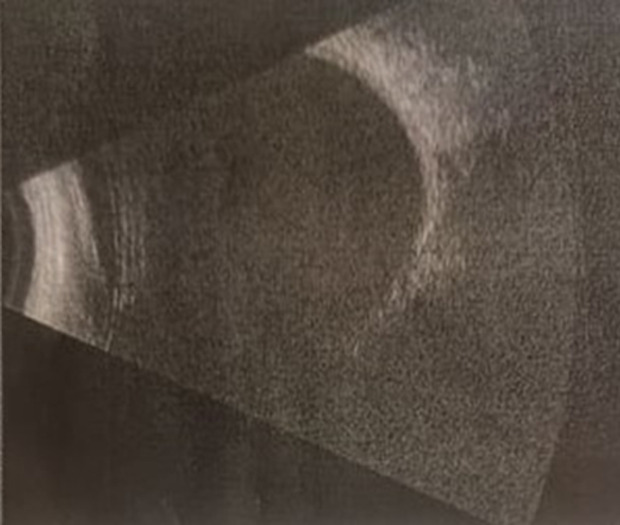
ultrasound B scan of the right eye showing a clear vitreous and no intraocular foreign body
